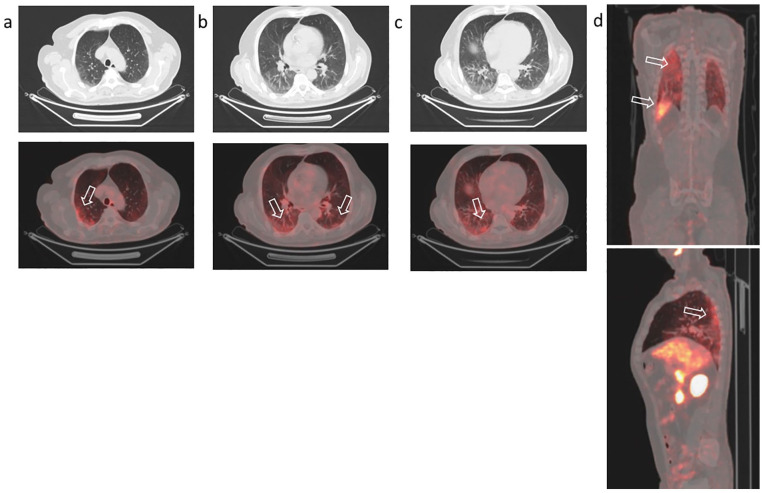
Positron emission tomography/computed tomography with ^68^Ga-PSMA to
